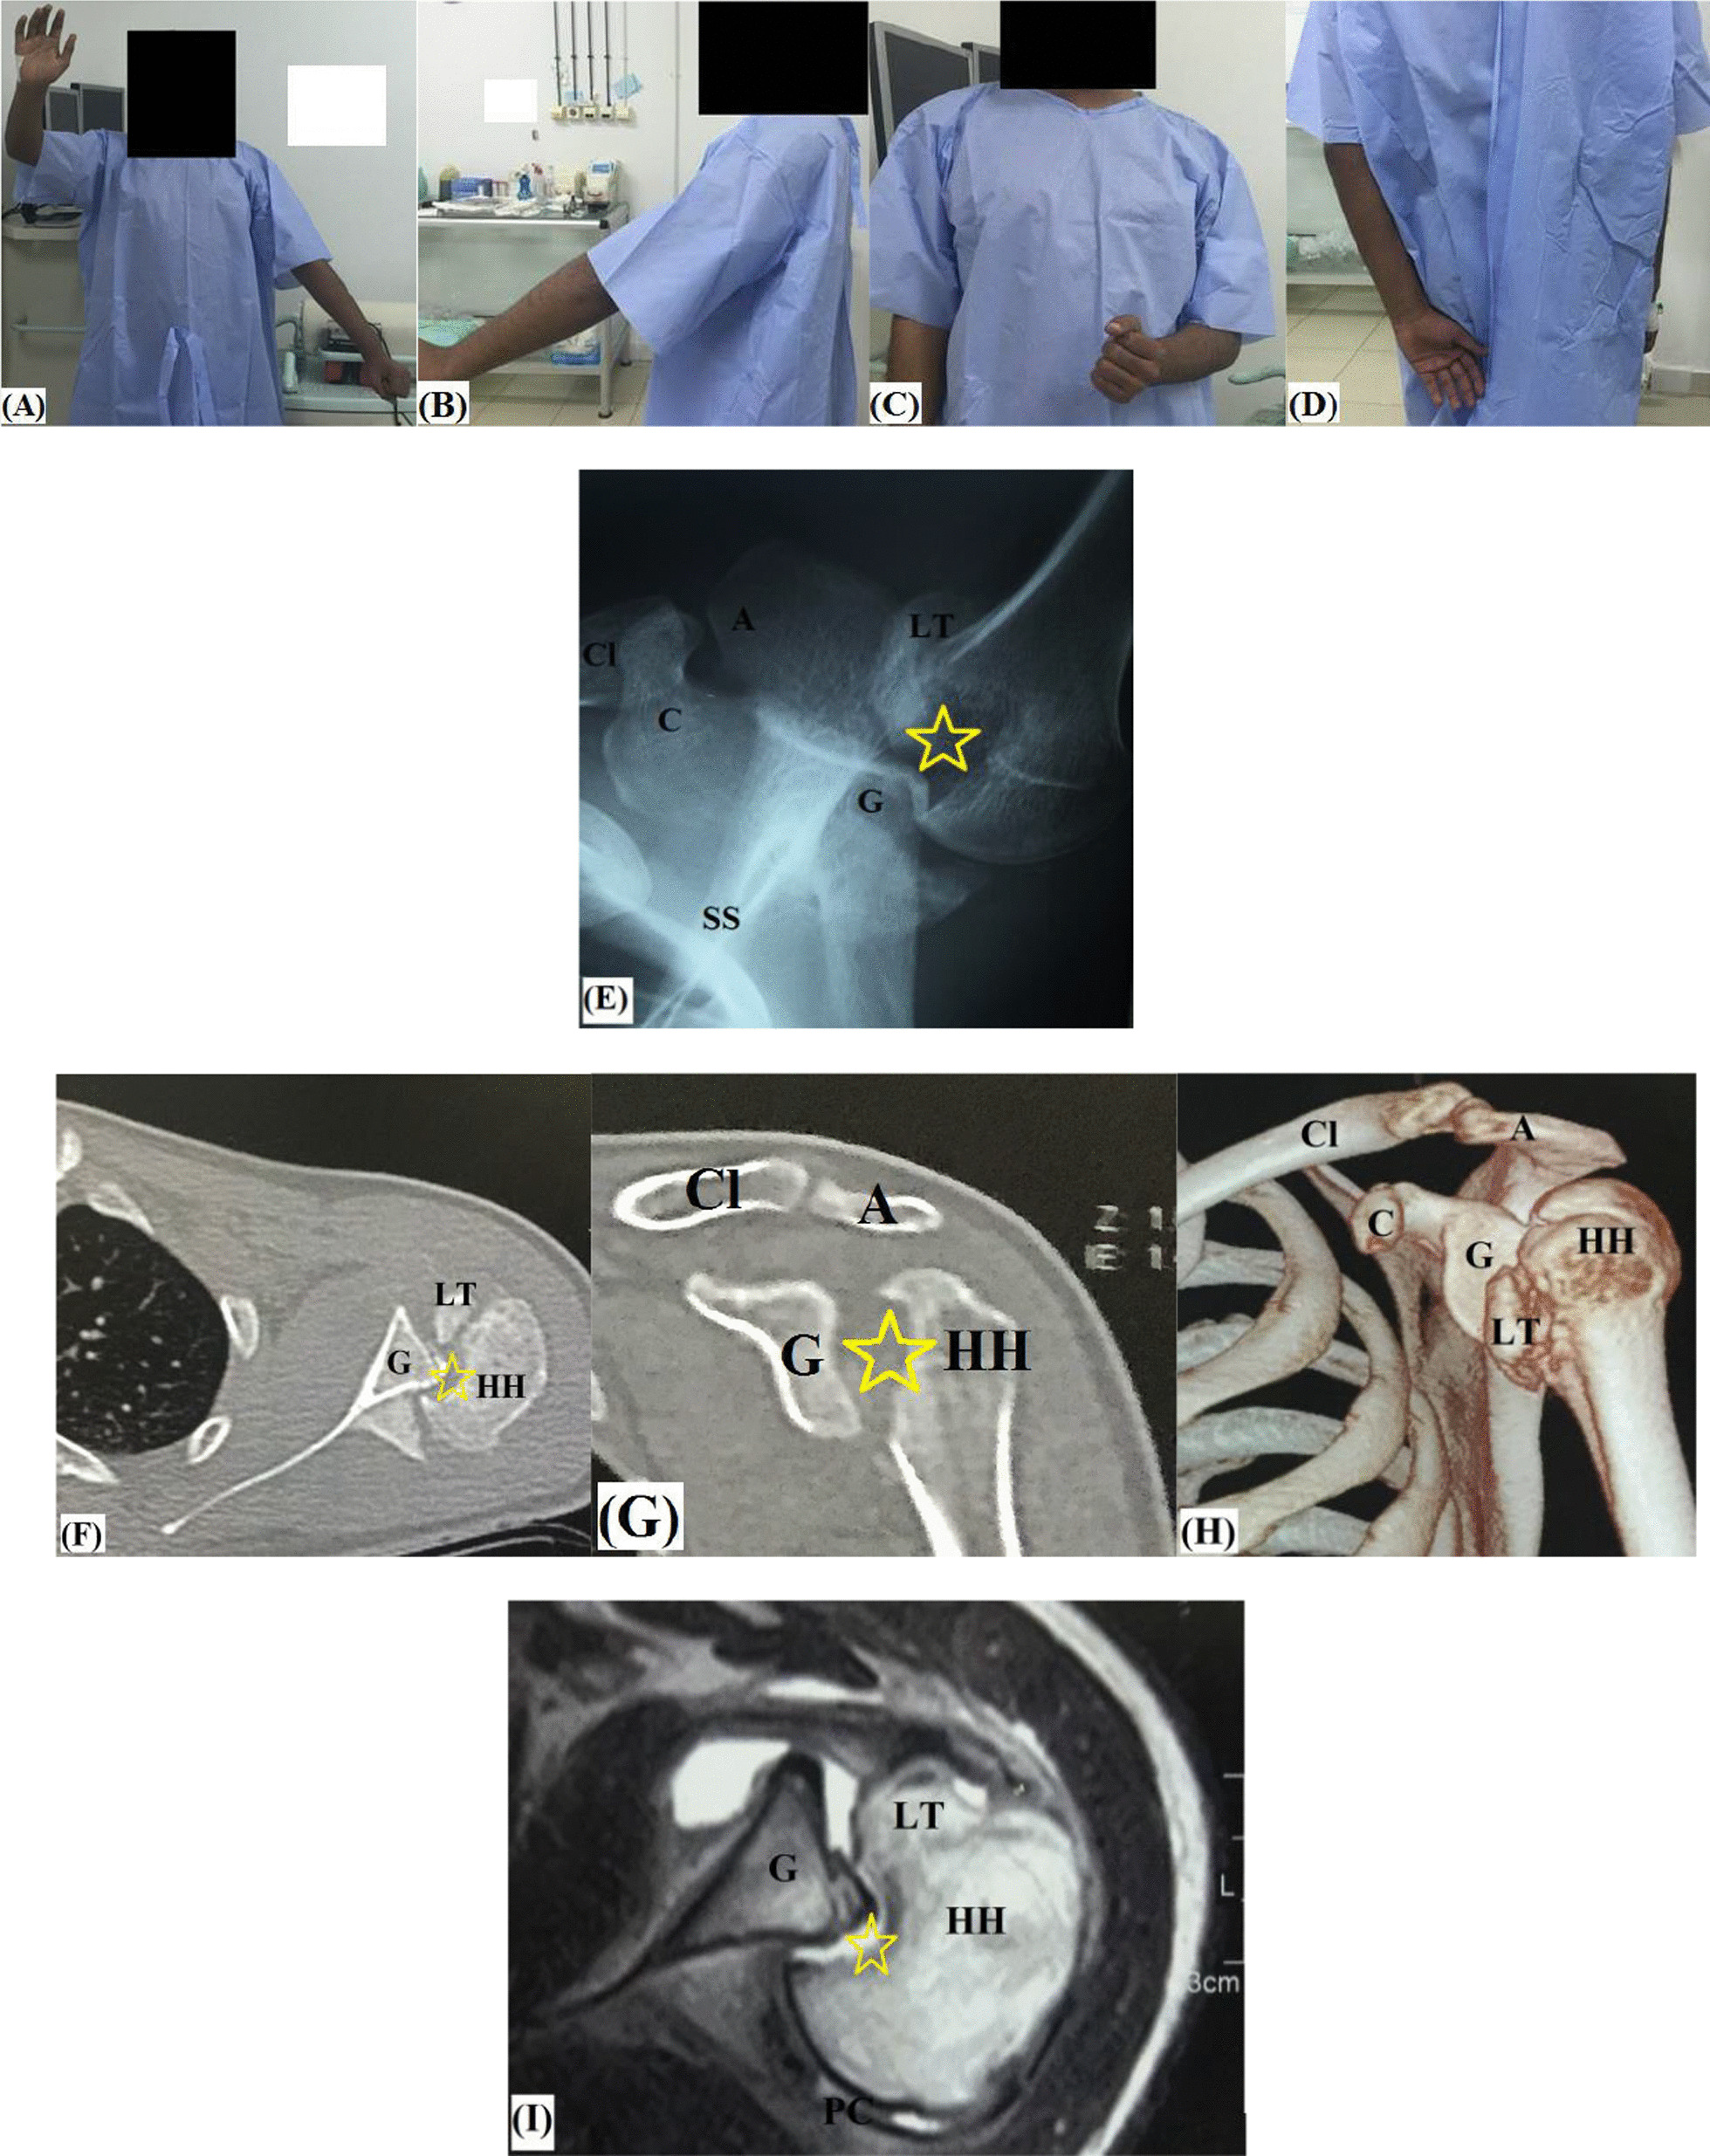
Preoperative assessment of chronic locked posterior GH dislocation (with yellow star-marked large reverse Hill-Sachs defect) in left shoulder; A–D active range of motion; E axillary X-ray view; F axial CT image; G coronal CT image; H 3D-reconstruction CT image; I axial MRI image; A, Acromion; C, Coracoid; Cl, Clavicle (lateral end); G, Glenoid; HH, Humeral head; LT, Lesser tuberosity; PC, Posterior capsule; SS, Scapular spine
